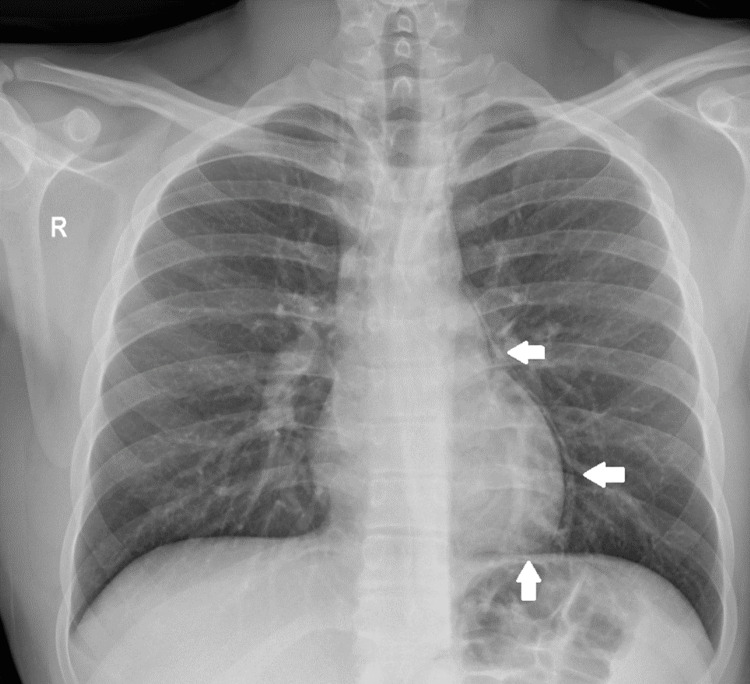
Chest X-ray in the upright position revealing a dark line extending from the level of the pulmonary artery to the apex of the heart and curving around its border (white arrows).
